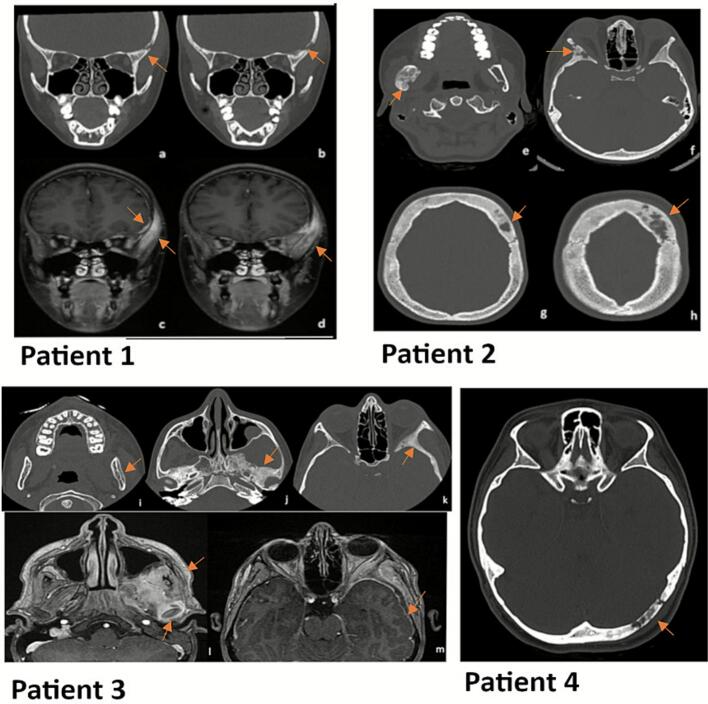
Patient 1: CT scan facial bones, coronal views, bone window: lytic left temporal bone lesion, cortical destruction (a,b). MRI of the face, coronal T1 weighted image with fat signal suppression and Gadolinium enhancement: left temporal bone lesion, adjacent lepto-meningeal thickening, muscular edema and temporo-mandibular synovitis (c,d) Patient 2: CT scan of facial bone and cranium, axial views, bone window: Lesion of the right ascending branch of the mandible, focal osteolysis, cortical destruction, osteosclerosis, unilamellar periosteal reaction (e). Multifocal bone lesions of the right orbit (f) and left frontal bone (g,h), focal osteolysis, cortical destruction and osteosclerosis. Patient 3: CT scan, axial cuts, bone window: Bone lesion of the left ascending mandible with unilamellar periosteal reaction (i). Multifocal lesions of the left sphenoidal bone (j) and orbit (k) with osteolysis, cortical erosion, osteosclerosis, and periosteal reaction. MRI: Lesion with leptomeningeal enhancement and temporo-mandibular synovitis (l, m). Patient 4: CT scan of the facial bones, axial views, bone window: lytic left occipital bone lesion with cortical erosion and adjacent osteosclerosis.
